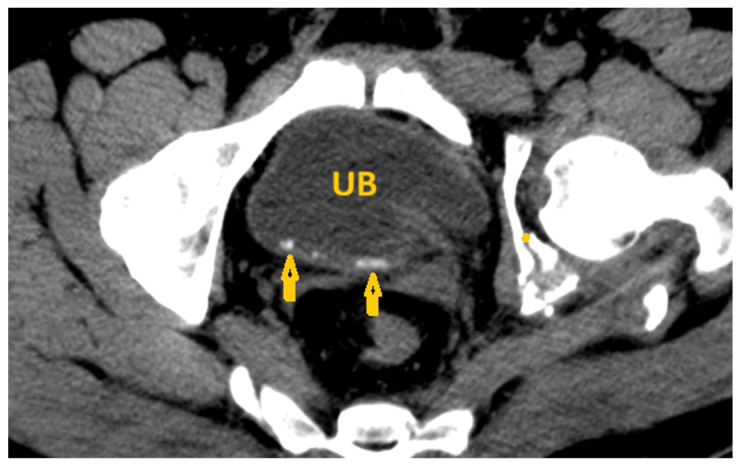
Selected non-contrast computed tomography axial pelvic image of a 36-year-old male patient shows a small linear calcification at the base of the urinary bladder (arrows), reflecting *Schistosoma* cystitis. UB: Urinary bladder.
